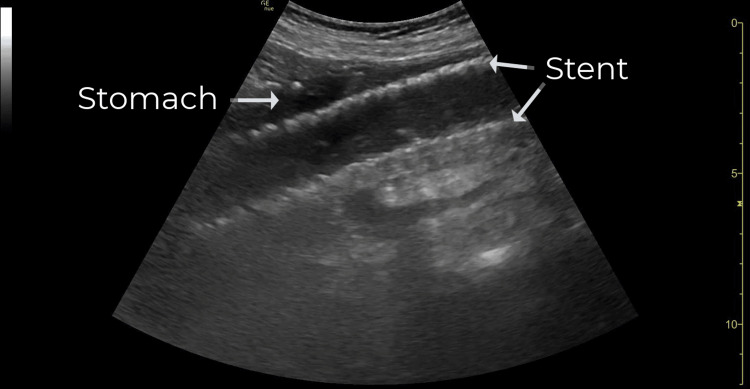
Point-of-care ultrasound of the stomach demonstrating a cylindrical form that was greater than 10 cm in length, echogenic, and fenestrated (long-axis view).
